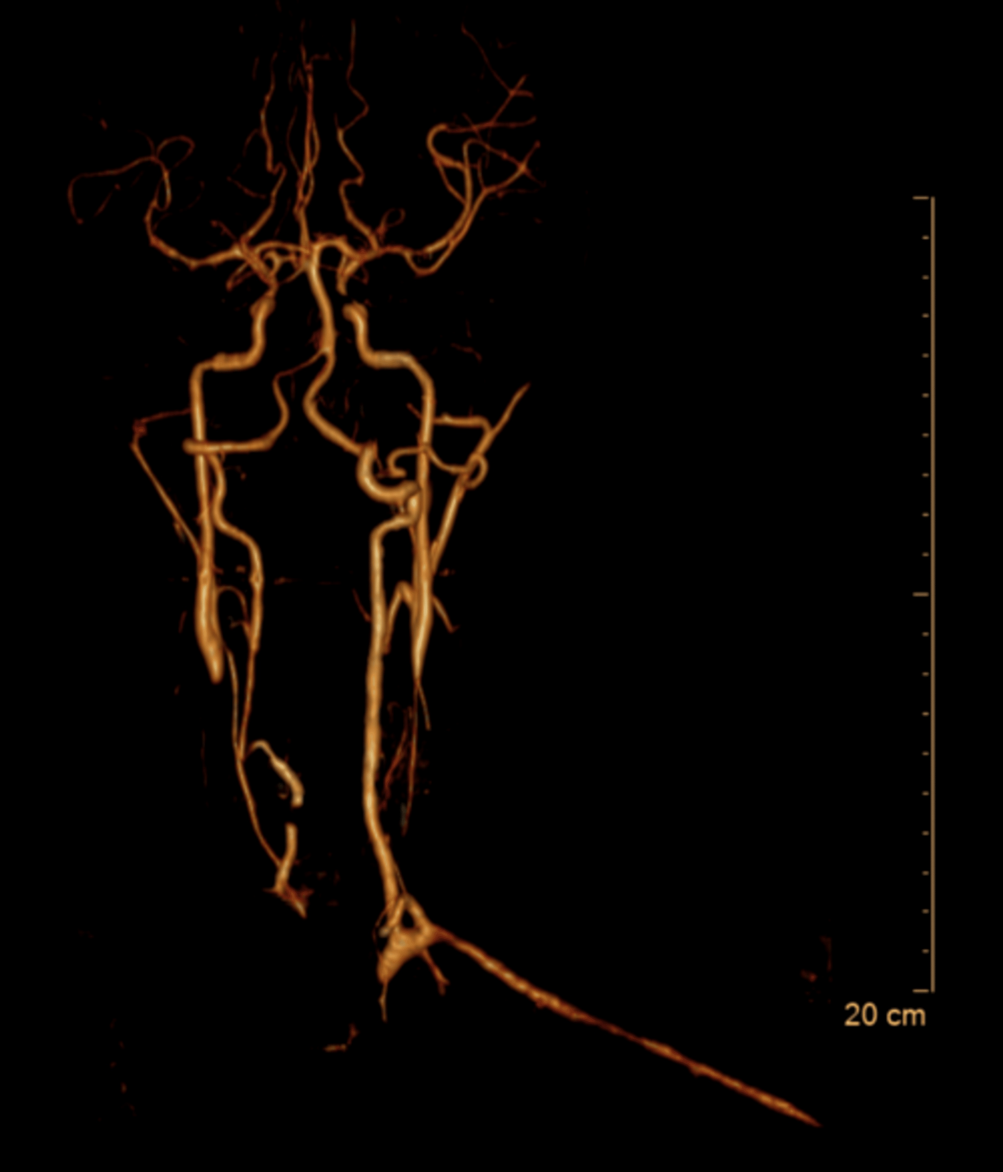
Three-dimensional computed tomography angiography revealing vascular truncation and tortuosity Three-dimensional reconstruction showing the marked truncation and pronounced tortuosity of the supra-aortic vessels, particularly involving the brachiocephalic trunk and carotid arteries, with relative preservation of distal intracranial circulation.
